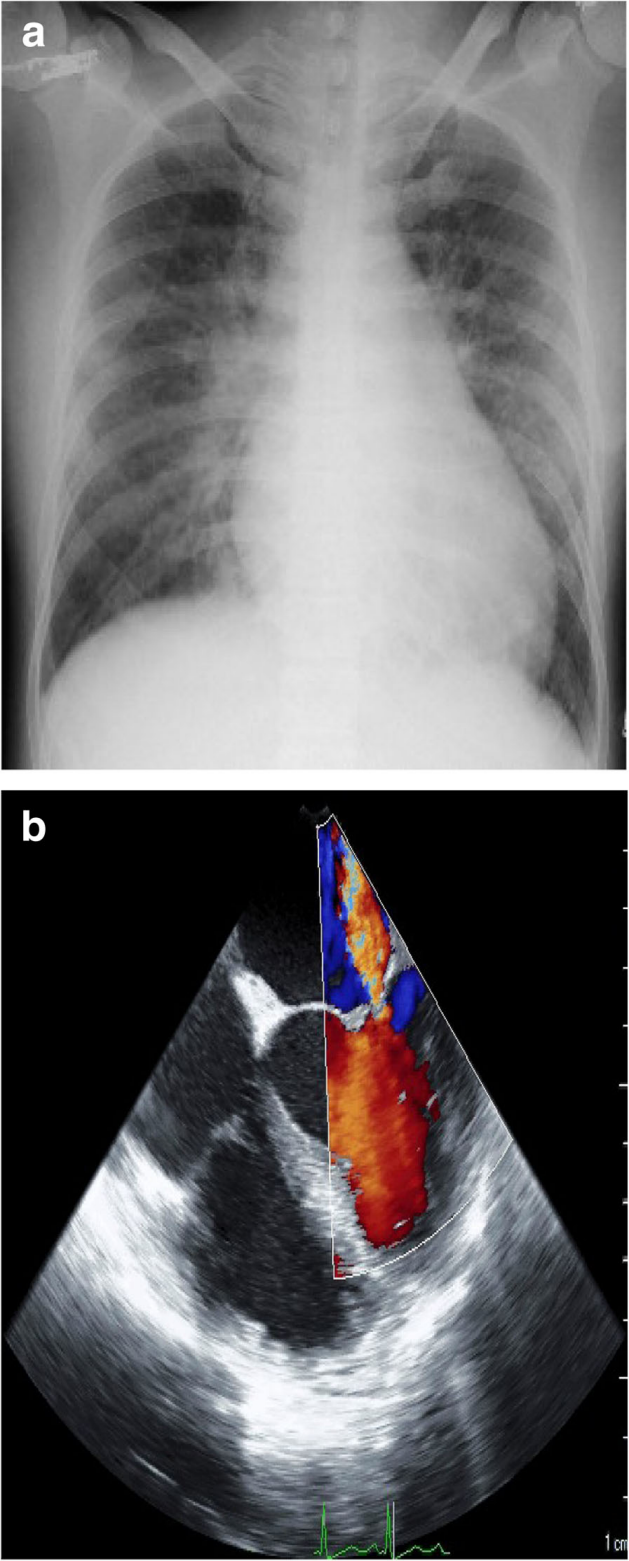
Examinations after exacerbation of dyspnea. a Chest radiograph showing cardiac enlargement and bilateral pulmonary infiltration. b Four-chamber view of a transesophageal echocardiogram showing severe mitral regurgitation
